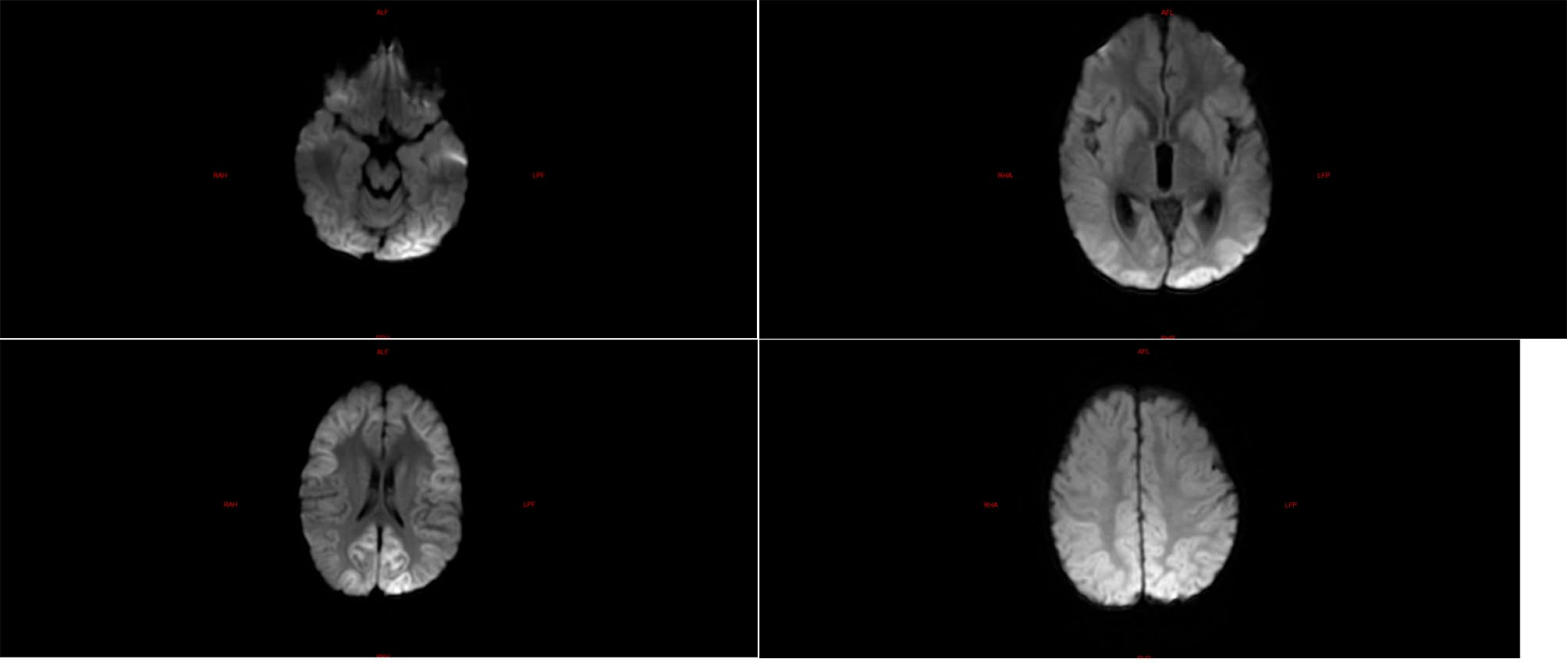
a, b, c, and d are brain MR DWI sequences of case 1, showing symmetrical diffuse hyperintensities in both supratentorial cerebral hemispheres, mainly involving the cortex and the basal ganglia. The patient was ultimately diagnosed with fulminant cerebral edema, with a rapid decline in consciousness to a GCS score of 3 within a short period. Despite stabilizing vital signs through aggressive treatment, the patient ultimately developed severe cognitive impairment and residual sequelae of limb paralysis
